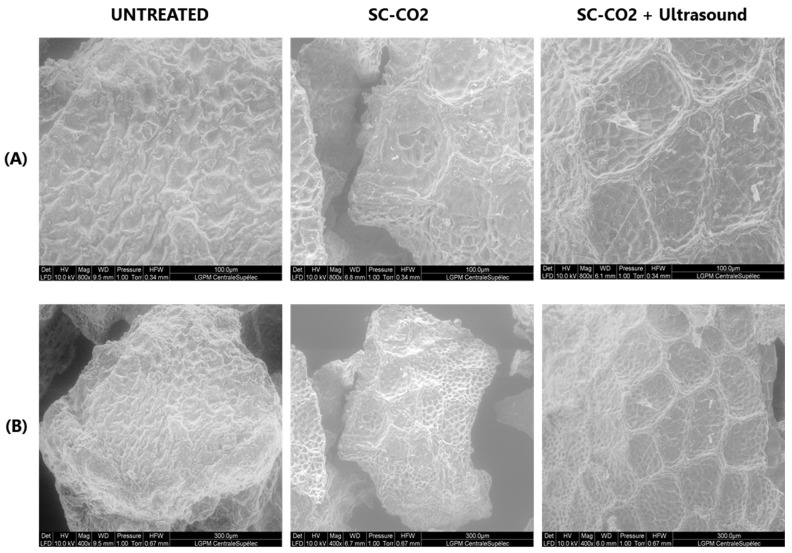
ESEM images of mustard seed meal subjected to SC-CO_2_ and ultrasound: (A) 400×–10 KV; (B) 800×–10 KV.
